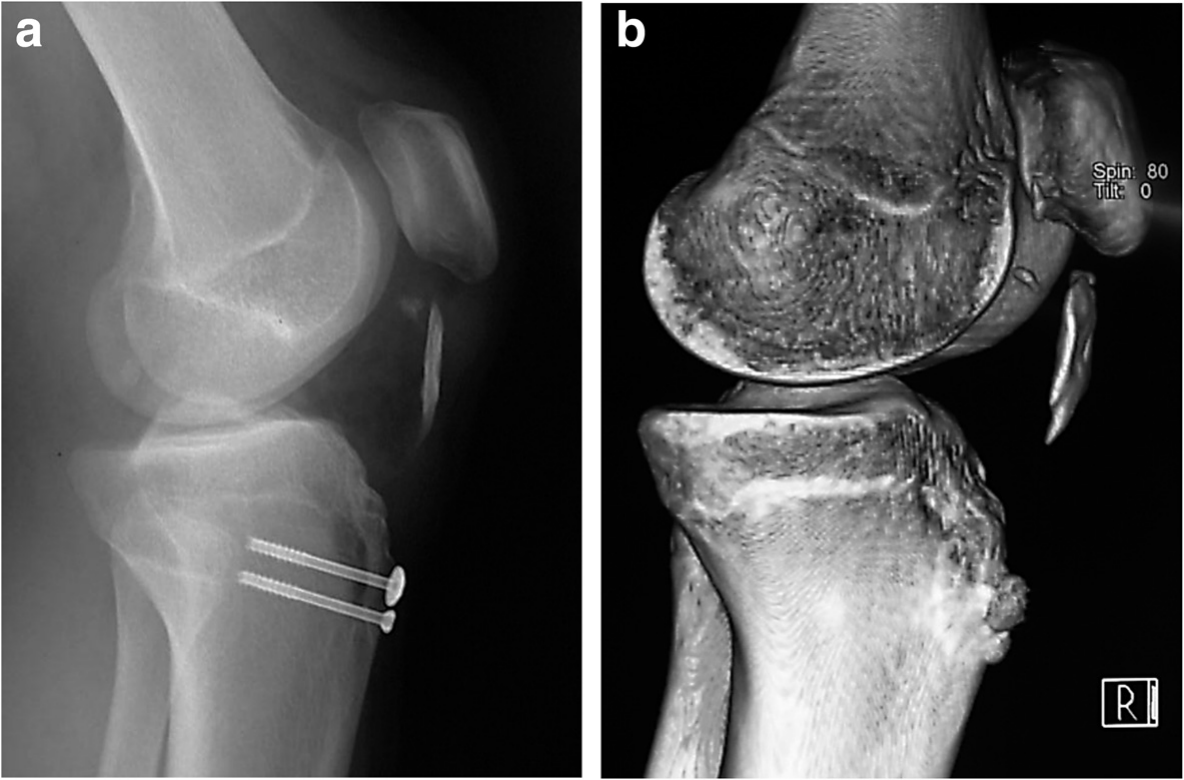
Lateral radiography (a) and computed tomography with three-dimensional reconstruction (b) of the right knee shows the presence of patella alta (Insall-Salvati index (Length of the patella/Length of the patella tendon) = 1.6) and ectopic calcification along the patellar tendon in case 1.
